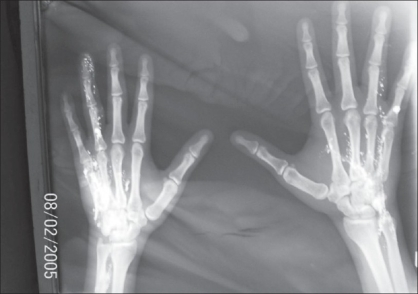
Patient - 1 Radiograph showing mercury deposit
